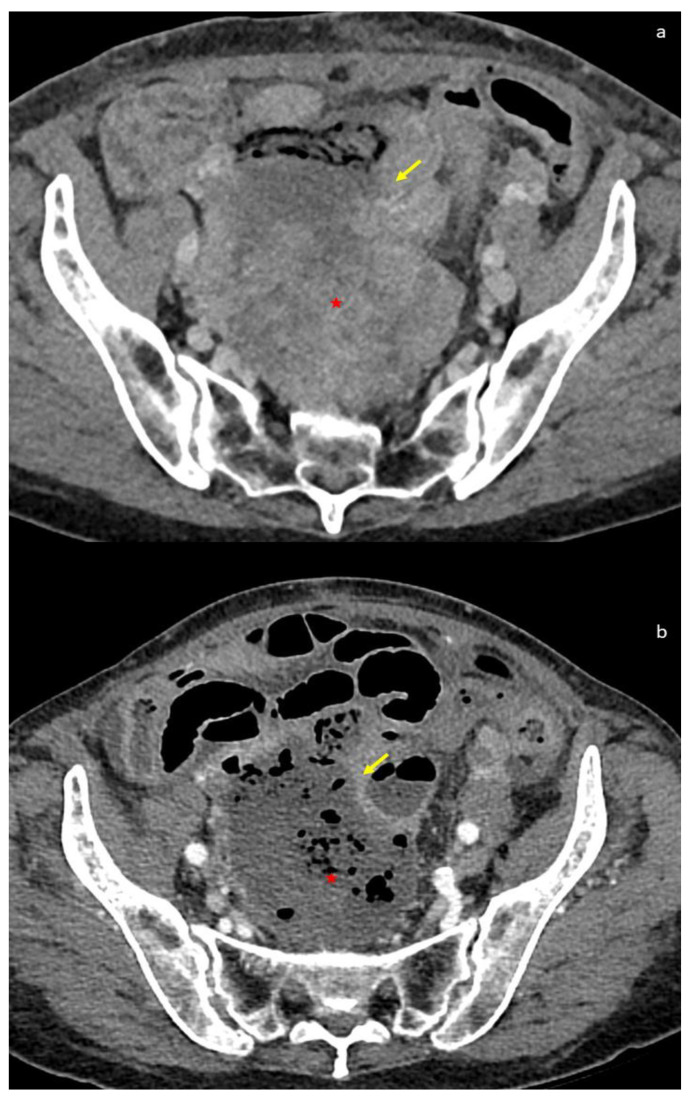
Our case: axial CT image depicting: GIST with attached ileal loop (yellow arrow) (a); GIST with signs of necrosis with attached ileal loop (red star) (b).
